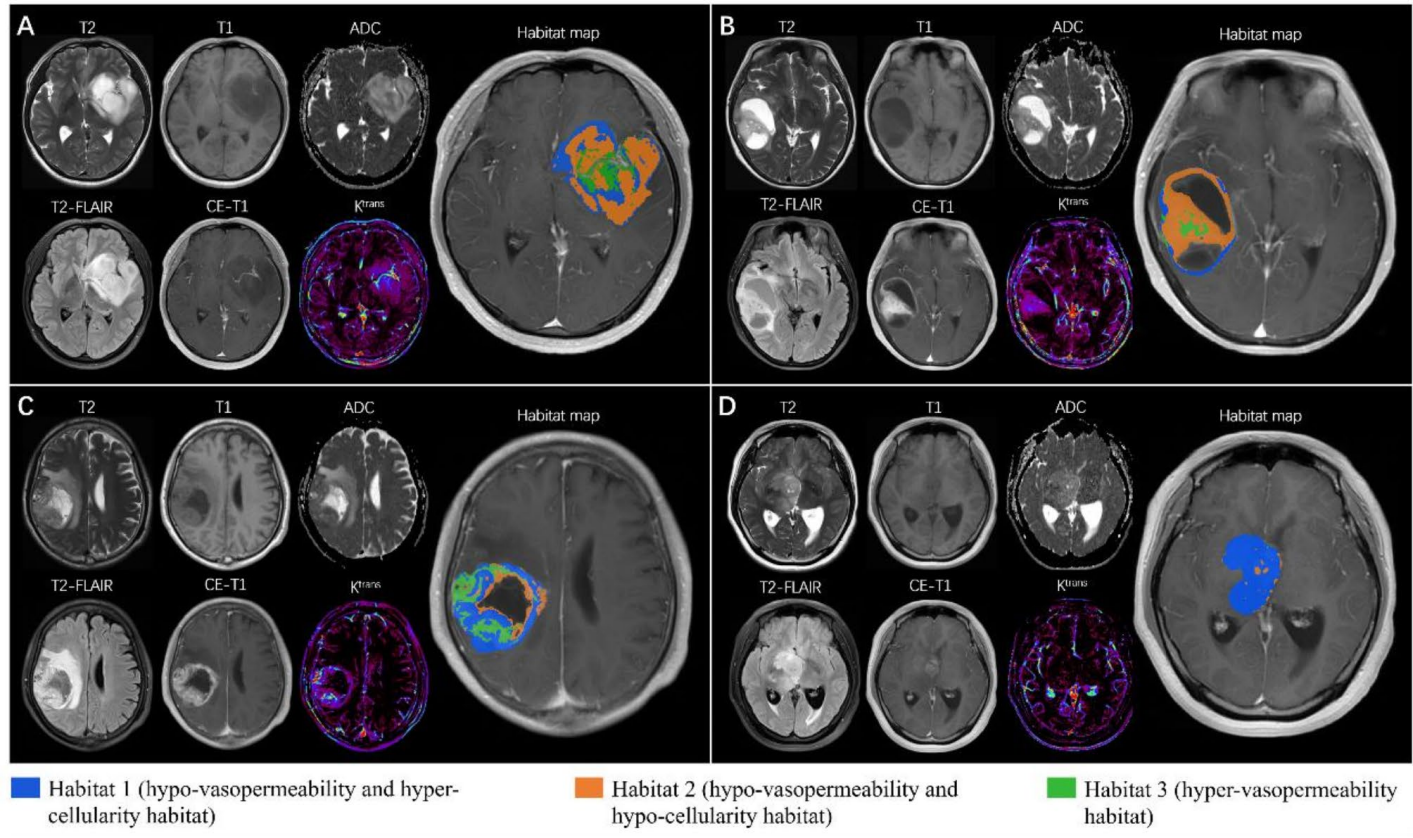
Demonstration of habitat segmentation slices in two patients with IDH mutant and wild-type gliomas. Spatial habitats are labeled with different colors. Contrast-enhanced T1-weighted imaging shows (A) a non-enhanced IDH mutant glioma, (B) an obviously enhanced IDH mutant glioma, (C) an obviously enhanced IDH wild-type glioma, and (D) a mildly enhanced IDH wild-type glioma
